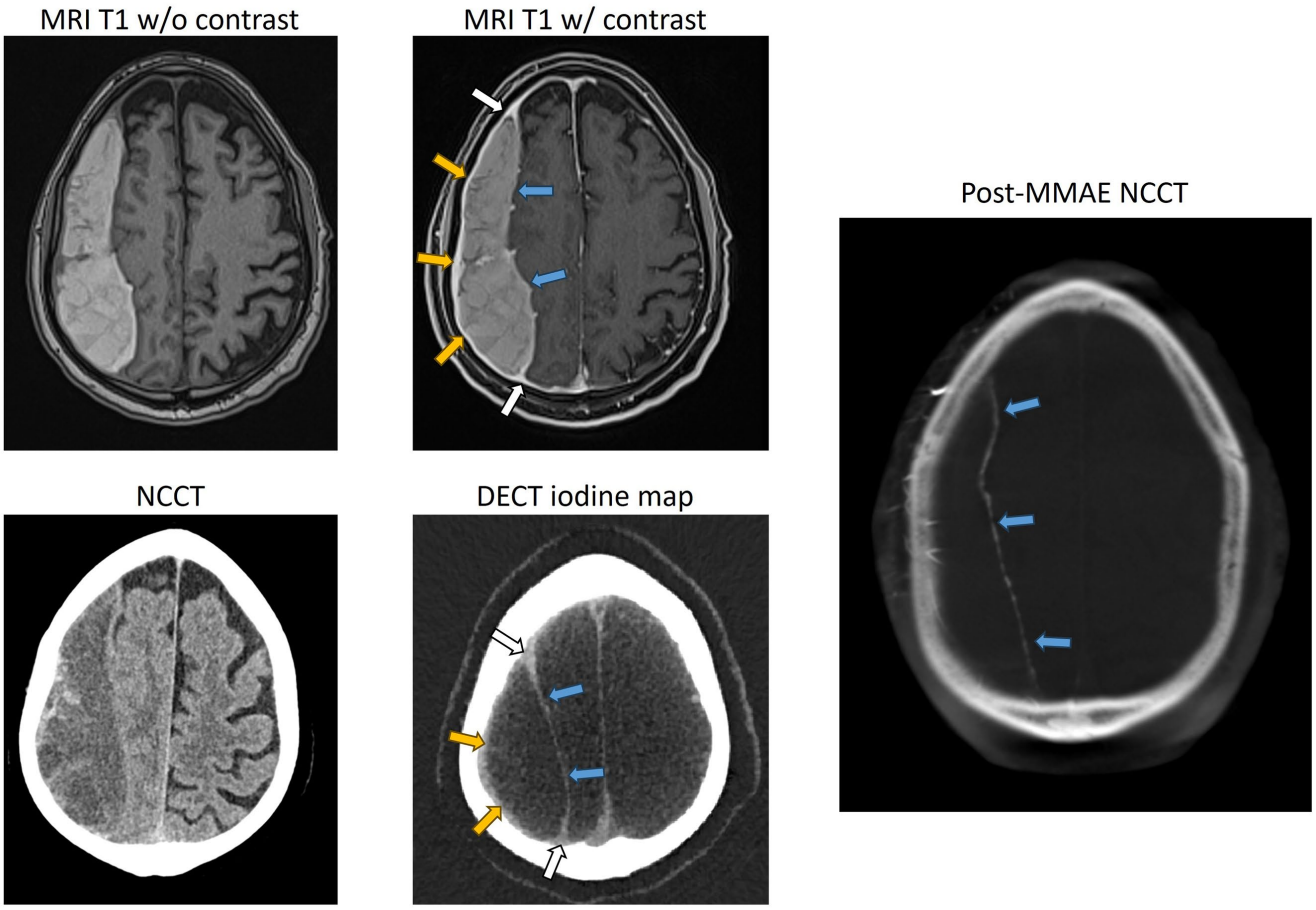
Representative case of a trabeculated cSDH, with contrasted MR, dual-energy CT, and DynaCT obtained during endovascular embolization showing the enhancement of the external membrane (yellow arrows), internal membrane (blue arrows), and “spandrel sign” (white arrows). Spandrel sign is defined as triangular thickened granulation tissue that forms the transition zone between the external and internal membranes. NCCT, non-contrast CT; DECT, dual-energy CT.
